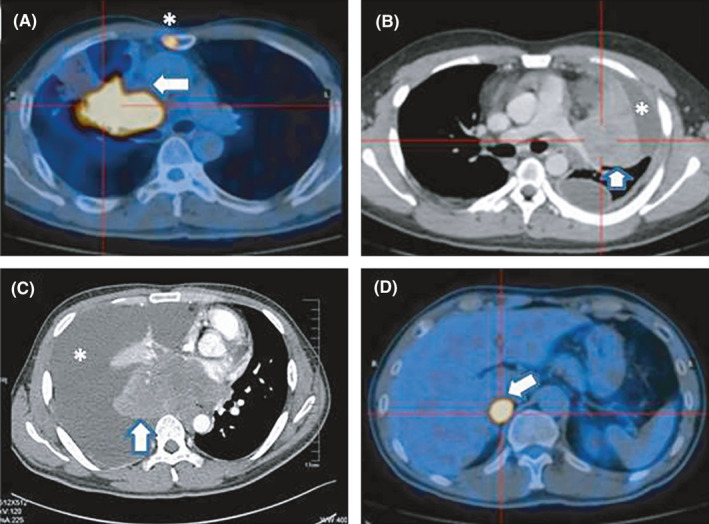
CT and PET‐CT images of pulmonary NUT midline carcinoma (NMC). (A) Pulmonary tumor in the right upper lobe (arrow) with SUV 18.6. Sternum metastasis is shown (asterisk). (B) Pulmonary tumor in the left upper lobe (arrow) with malignant pleural effusion (asterisk). (C) Pulmonary tumor in the right low lobe (arrow) with malignant pleural effusion (asterisk). (D) Adrenal metastasis with SUV 17.8 (arrow)
